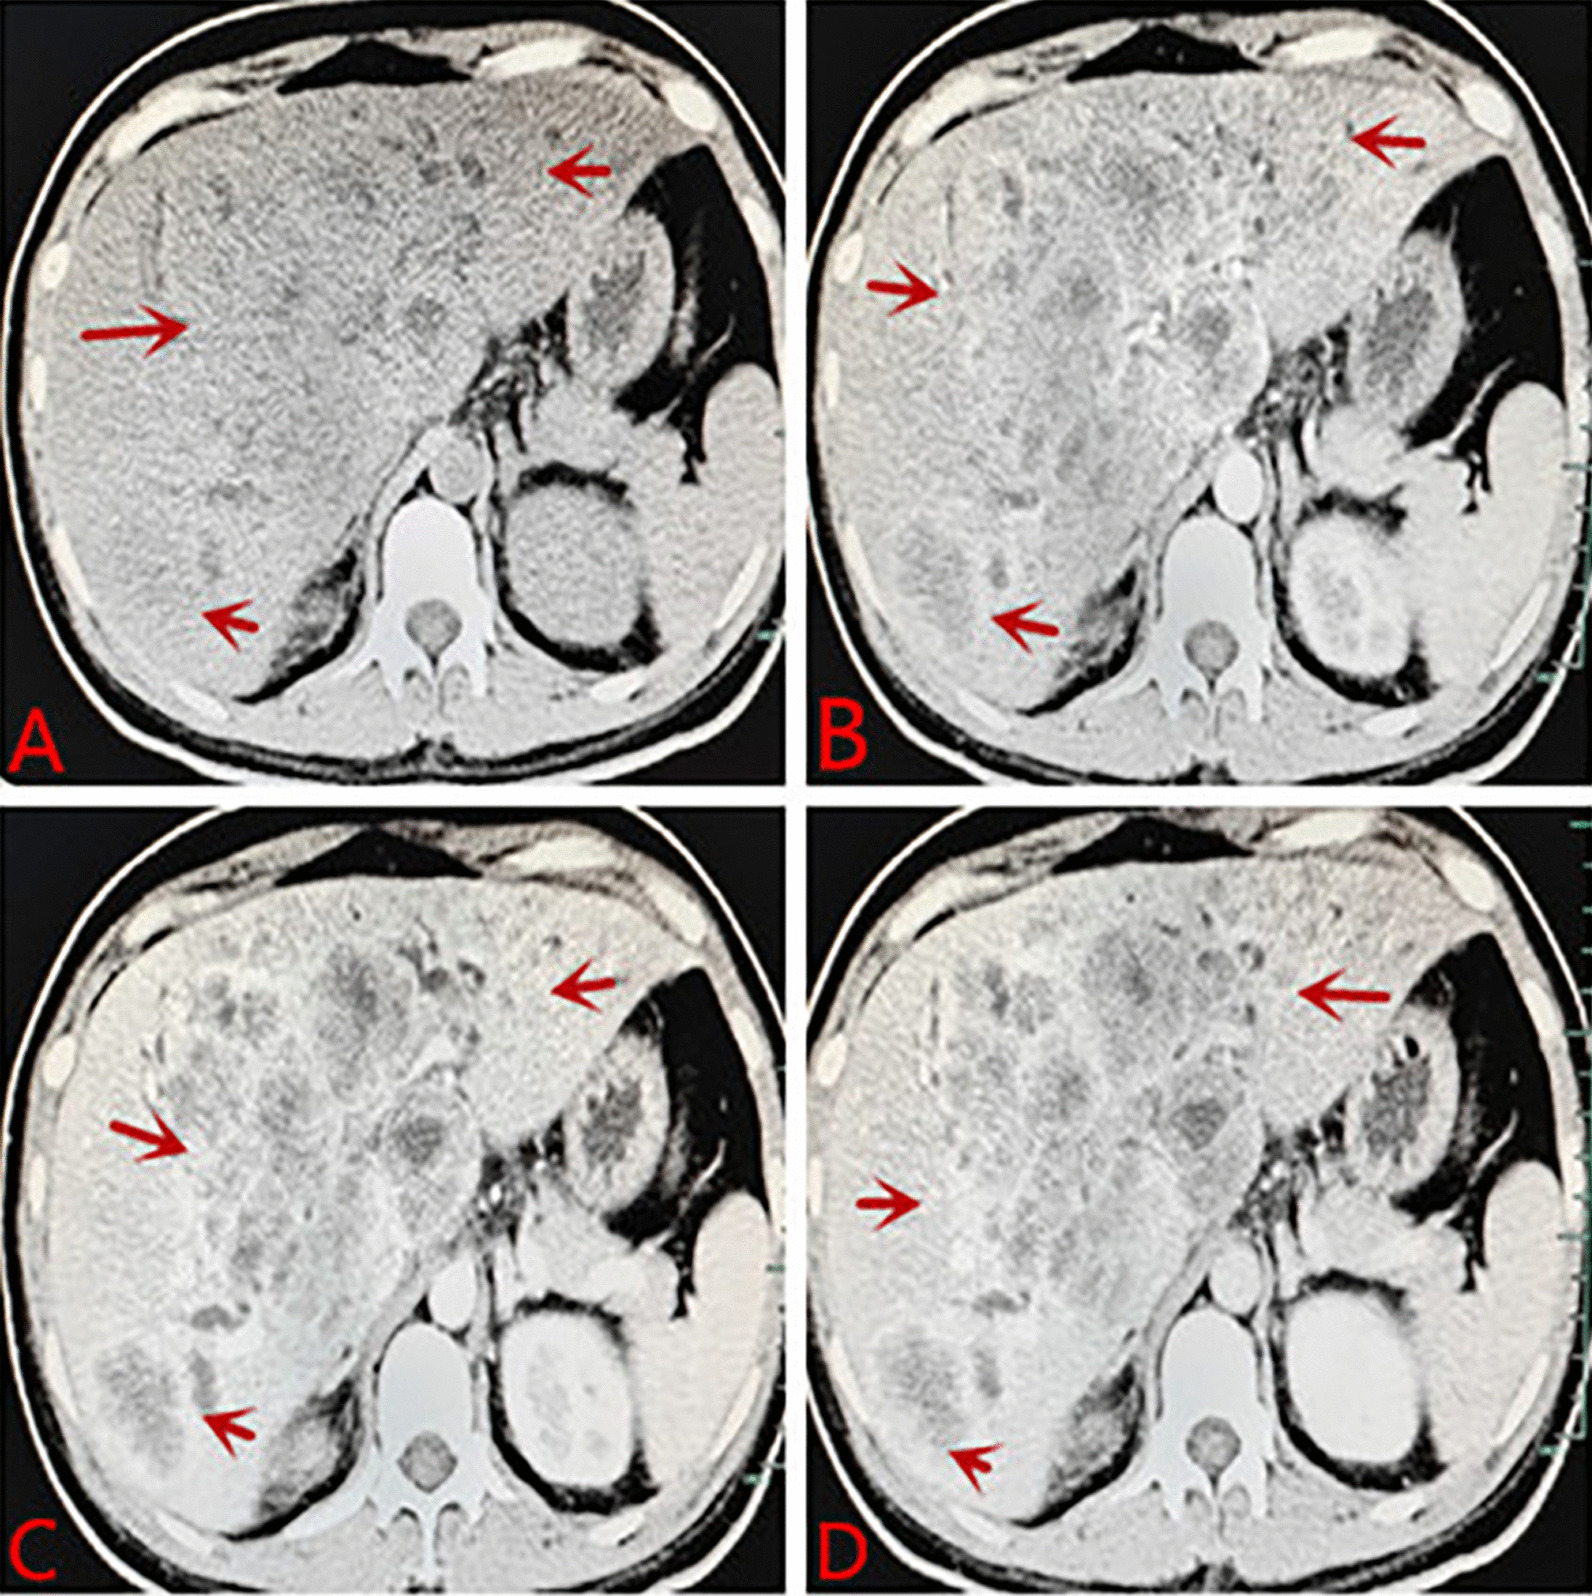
A Plane CT scan indicates less regular shape of liver, diffuse mass-like and nodular slightly hypodense lesions, some of which were fused. B Contrast-enhanced CT: significant ring enhancement in the arterial phase. C, D Continuous ring enhancement in the venous and delayed phases, local wall thinning and irritability in the right and left branches of the portal vein. Consider malignant tumors, a high possibility of hepatocellular carcinoma or cholangiocarcinoma, with local invasion of the right and left branches of the portal vein, hilar lymphadenopathy, and a small amount of ascites
